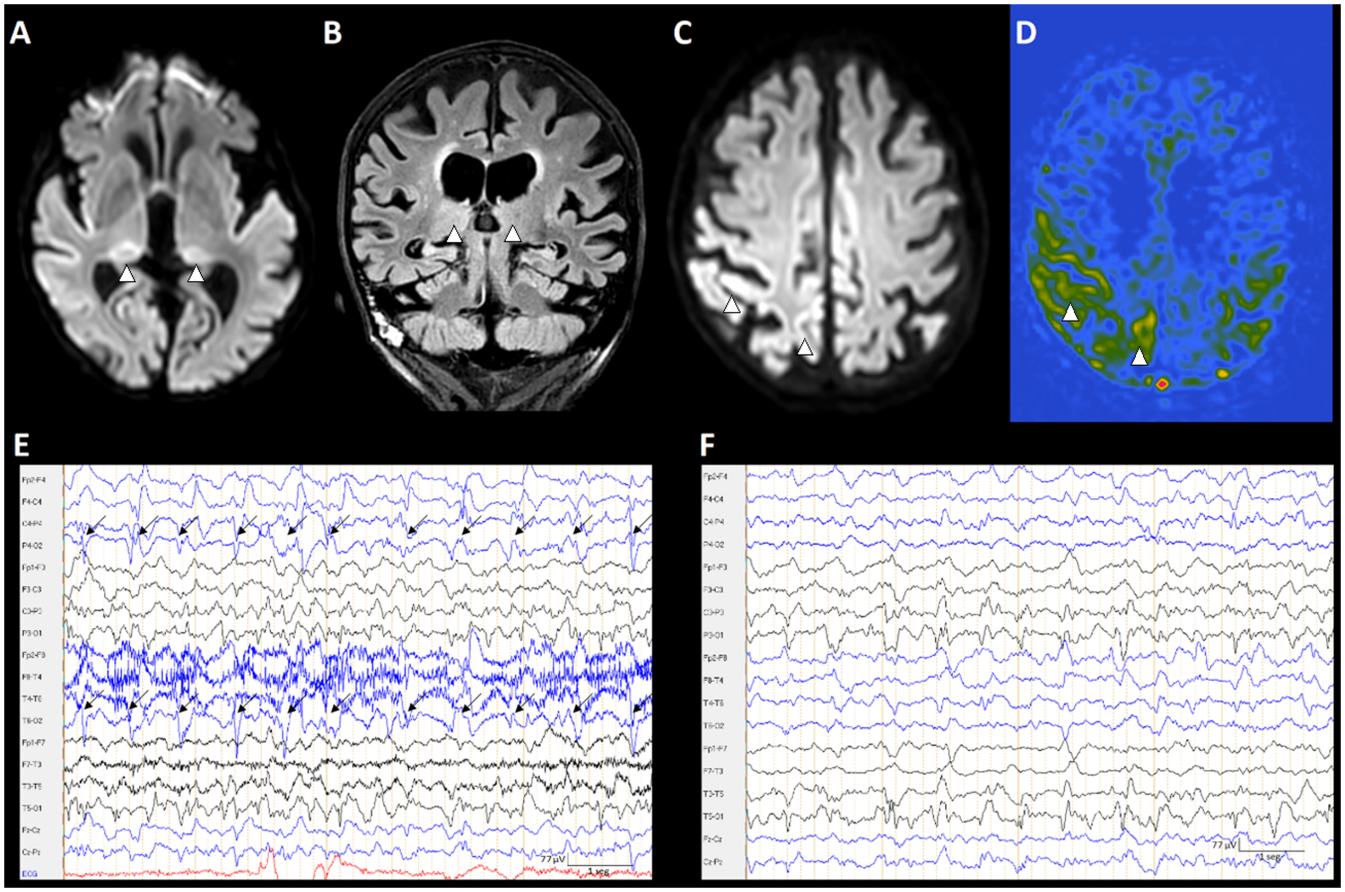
Patient with a focal to bilateral tonic–clonic seizure lasting 3 min, followed by decreased level of consciousness progressing to coma. MRI demonstrated typical PMA, and EEG findings were consistent with IIC. Bilateral pulvinar involvement with concordant ASL hyperperfusion and associated EEG changes. Top row: MRI (A–D). Bottom row: EEG (E,F). (A) Axial diffusion-weighted imaging (DWI) demonstrates bilateral diffusion restriction in the pulvinar nuclei (arrowheads). (B) Coronal FLAIR shows bilateral pulvinar hyperintensity; due to slight asymmetry of the coronal slice angulation, the right pulvinar appears mildly brighter than the left (arrowheads). (C) Axial DWI reveals laminar cortical diffusion restriction in the right parietal cortex (arrowheads). (D) Arterial spin labeling (ASL) shows focal hyperperfusion spatially concordant with the diffusion-restricted cortex in (C) (arrowheads). (E) EEG demonstrates lateralized periodic discharges with superimposed fast activity (LPDs + F; 1.1 Hz) over the right posterior quadrant (arrows). (F) EEG after treatment. EEGs were displayed using a 1 Hz low-frequency filter, 70 Hz high-frequency filter, 50 Hz notch filter, and a sensitivity of 7 μV/mm.
